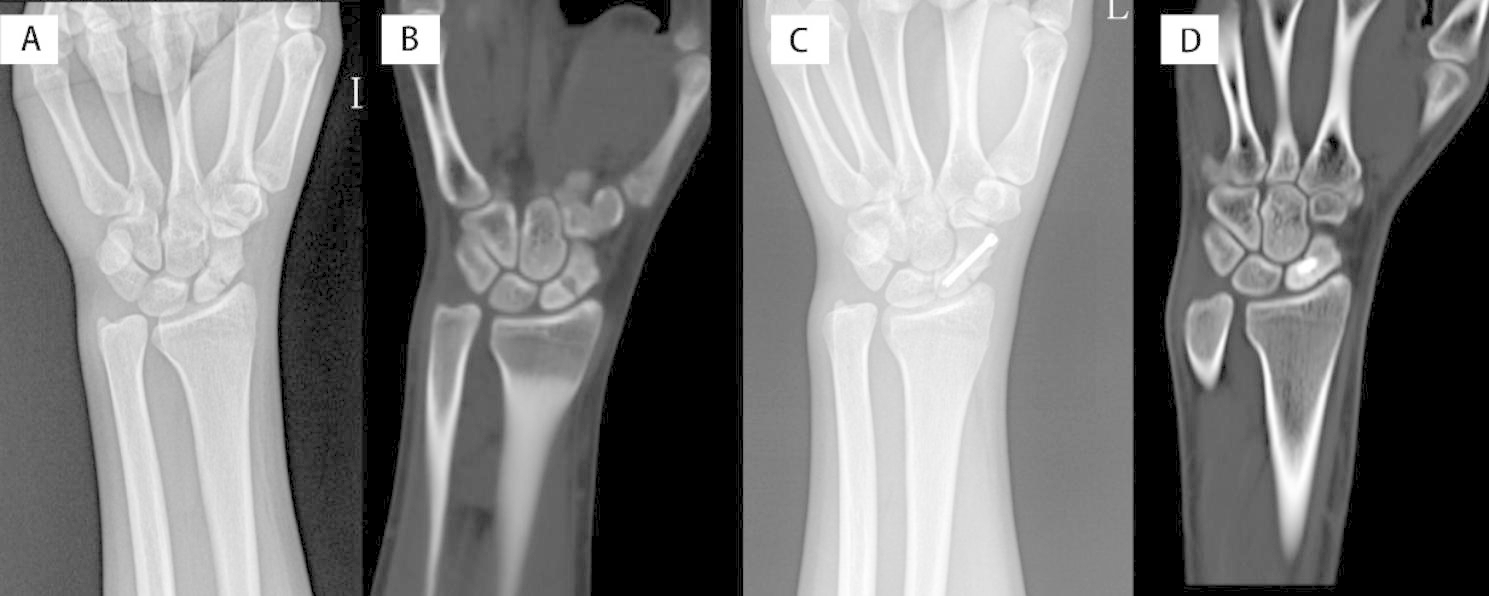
A-B. 19 male patient with scaphoid nonunion in the left hand, with time passed from trauma of 10 months, Preoperative radiographs. Figure 2 C. Immediately radiographs after surgery. Figure 2D. 3 month postoperative radiographs
